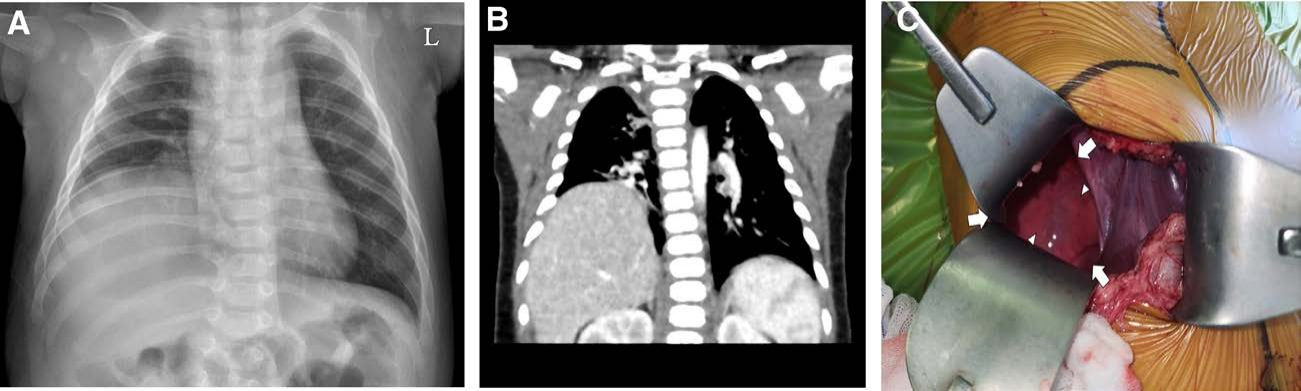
Preoperative imaging and intraoperative findings in case 3. (A) Chest x-ray reveals a focal opacity in the right lower lung zone with an elevated right diaphragmatic margin, initially suggestive of pneumonia. (B) CT scan demonstrates the liver herniating into the thoracic cavity, indicating a diaphragmatic hernia or eventration. (C) Intraoperative findings show a diaphragmatic defect (arrow), with lung tissue visible beyond a thin sac (arrowhead). CT = computed tomography.
